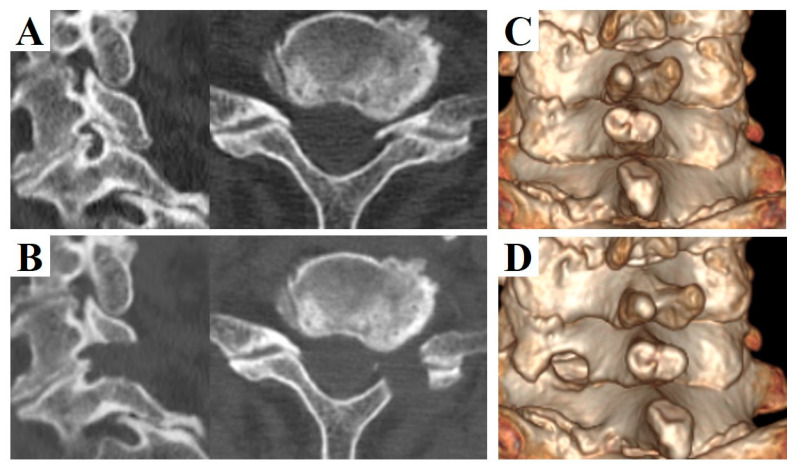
Pre- and postoperative computed tomography (CT) images of a 73-year-old man who underwent left C6/7 full-endoscopic cervical foraminotomy (FECF). (A) Preoperative sagittal (left) and axial (right) CT images. (B) Postoperative sagittal (left) and axial (right) CT images. (C) Pre- and (D) postoperative 3-dimensional CT images. Note that the dorsal part of the vertebral foramen was removed after FECF.
